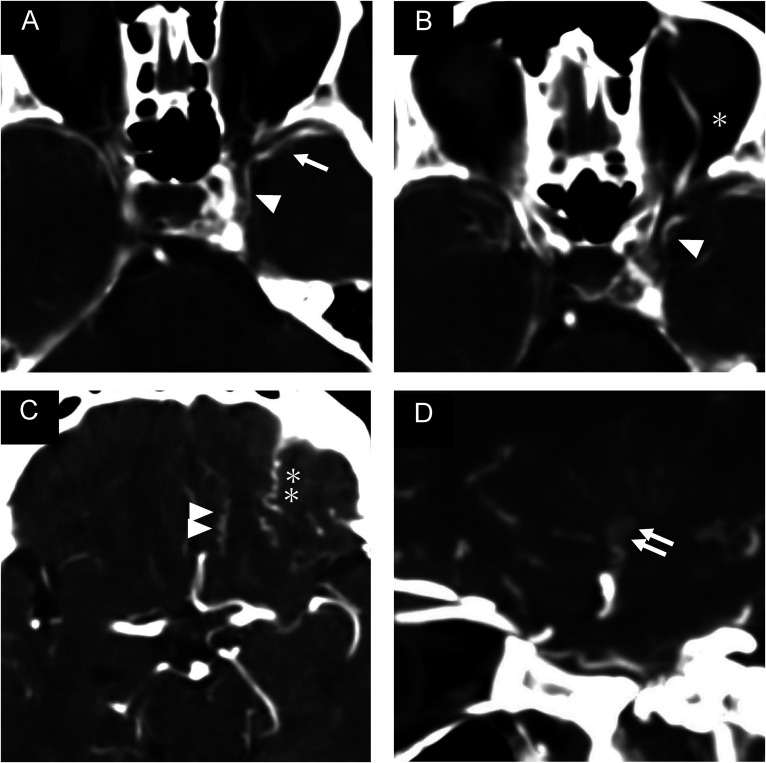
Pre-procedural head computed tomography angiogram showing early contrast opacification of the left cavernous sinus and left superficial middle cerebral vein (single arrow) connected to the laterocavernous sinus (single arrowhead) (A). Multidirectional drainage routes including the superior ophthalmic vein (B, single asterisk), olfactory vein (C, double arrowhead), orbitofrontal vein (C, double asterisk), and inferior striate vein (D, double arrow) can be observed.
